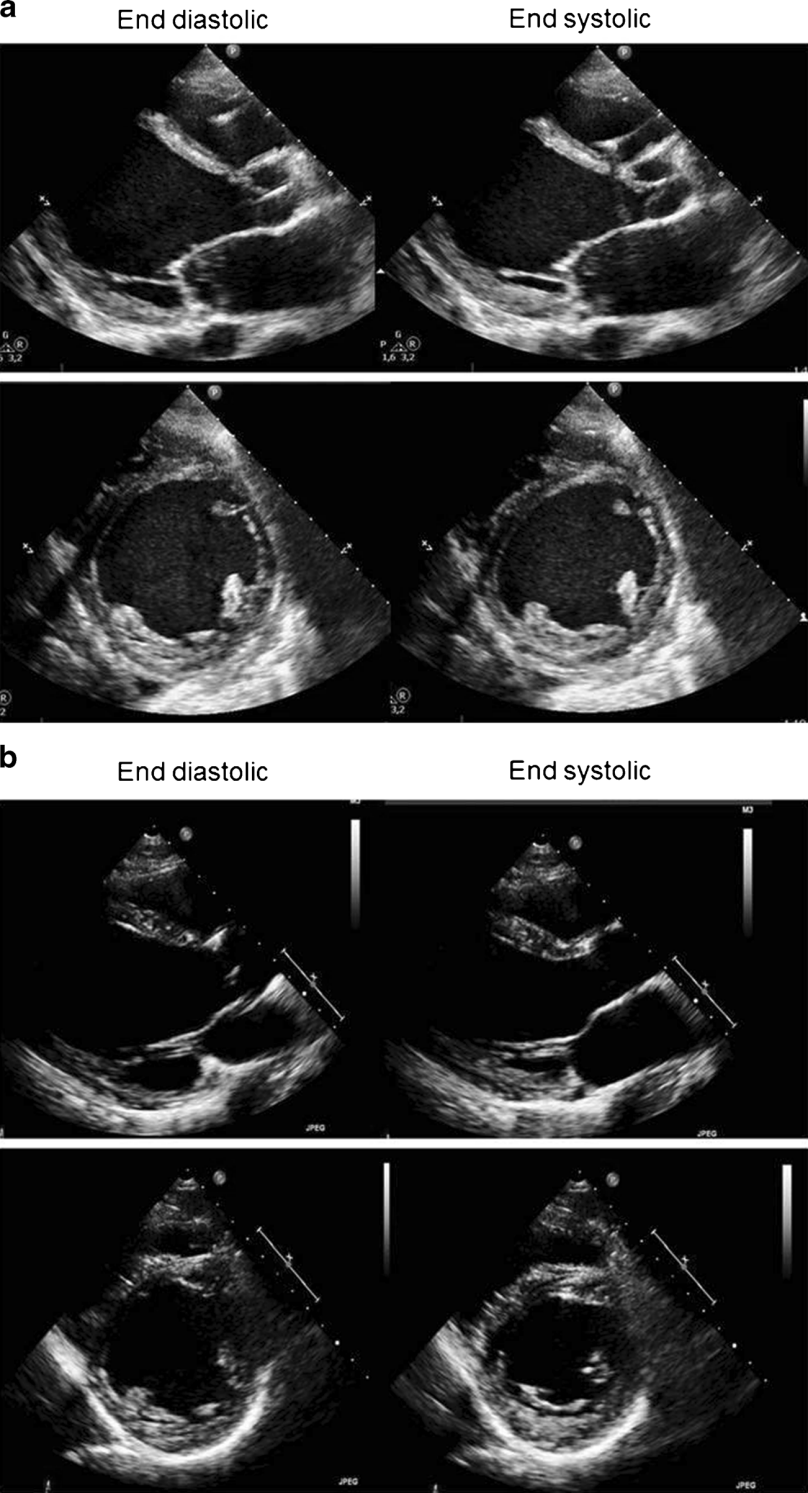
a Initial phase (week 1). Transthoracic echocardiographic images representative of case 1; diastolic (*left*) and systolic (*right*) still frames from parasternal long axis, short axis views. Side box. LVEDD 68 mm. LVESD 62 mm. Estimated LVEF 10 %. b After ECMO and heart failure medical treatment (6 months). Transthoracic echocardiographic images representative of case 1; diastolic (*left*) and systolic (*right*) still frames from parasternal long axis, short axis views. Side box. LVEDD 65 mm. LVESD 44 mm. Estimated LVEF 35–40 %
